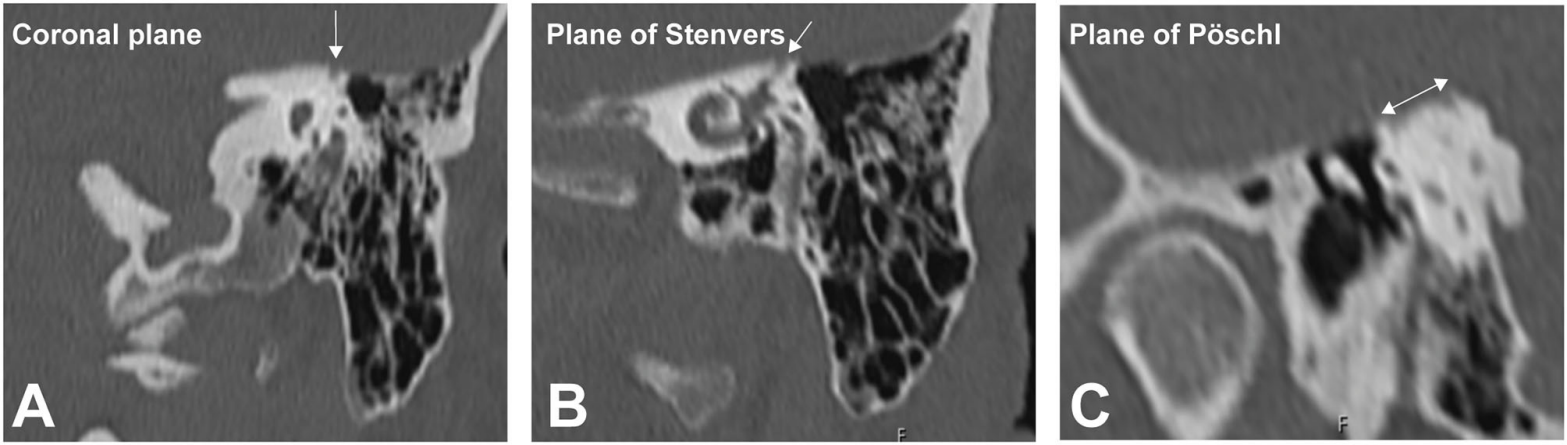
High-resolution temporal bone imaging CT (slice thickness 0.6 mm). The semicircular canal (SCC) dehiscence is indicated by the white arrows in the (A) coronal plane, (B) plane of Stenvers, and (C) plane of Pöschl.
